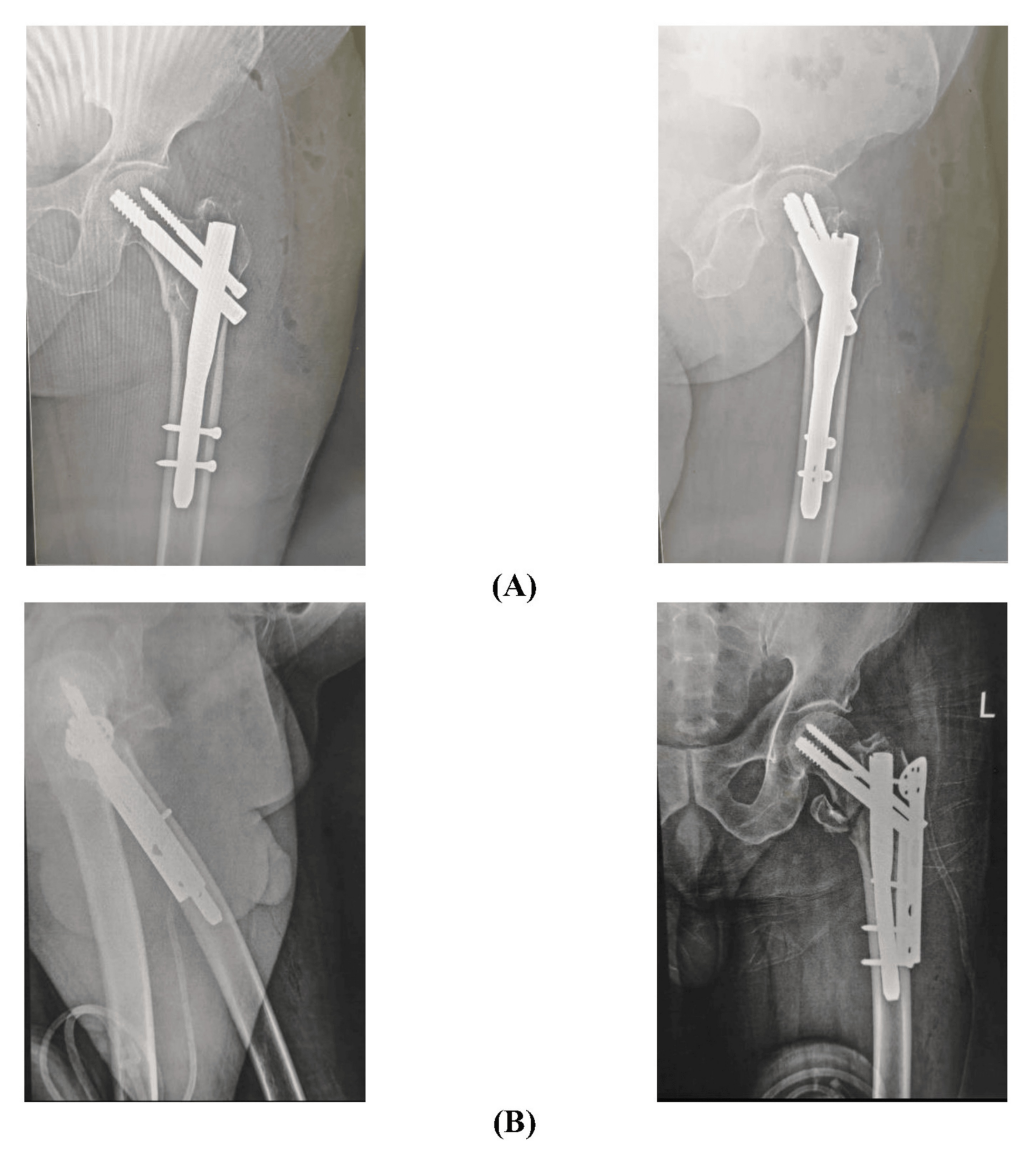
(A) Postoperative X-rays showing intertrochanteric femur fracture managed by PFN only, and (B) postoperative X-rays showing intertrochanteric femur fracture managed by PFN with TBP augmentation PFN: proximal femoral nail; TBP: trochanteric buttress plate
